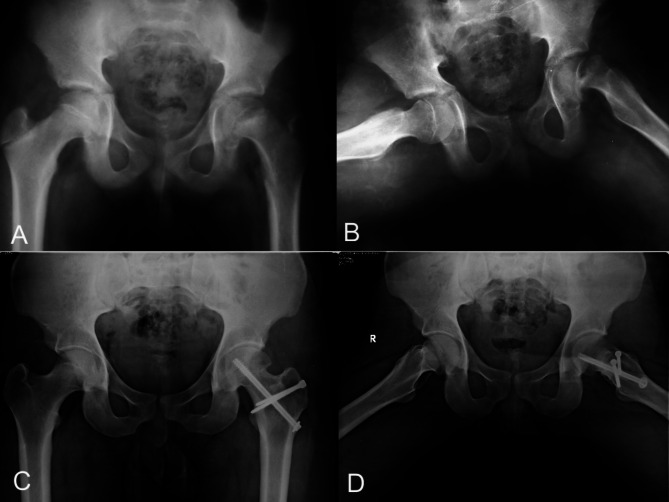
A) and B): Preoperative radiographs of a 14-year-old male patient with acute on top of chronic SCFE. C) and D) Final follow-up x-rays done ten years after the surgery show no evidence of AVN or hip osteoarthritis with complete healing of the osteotomy site
